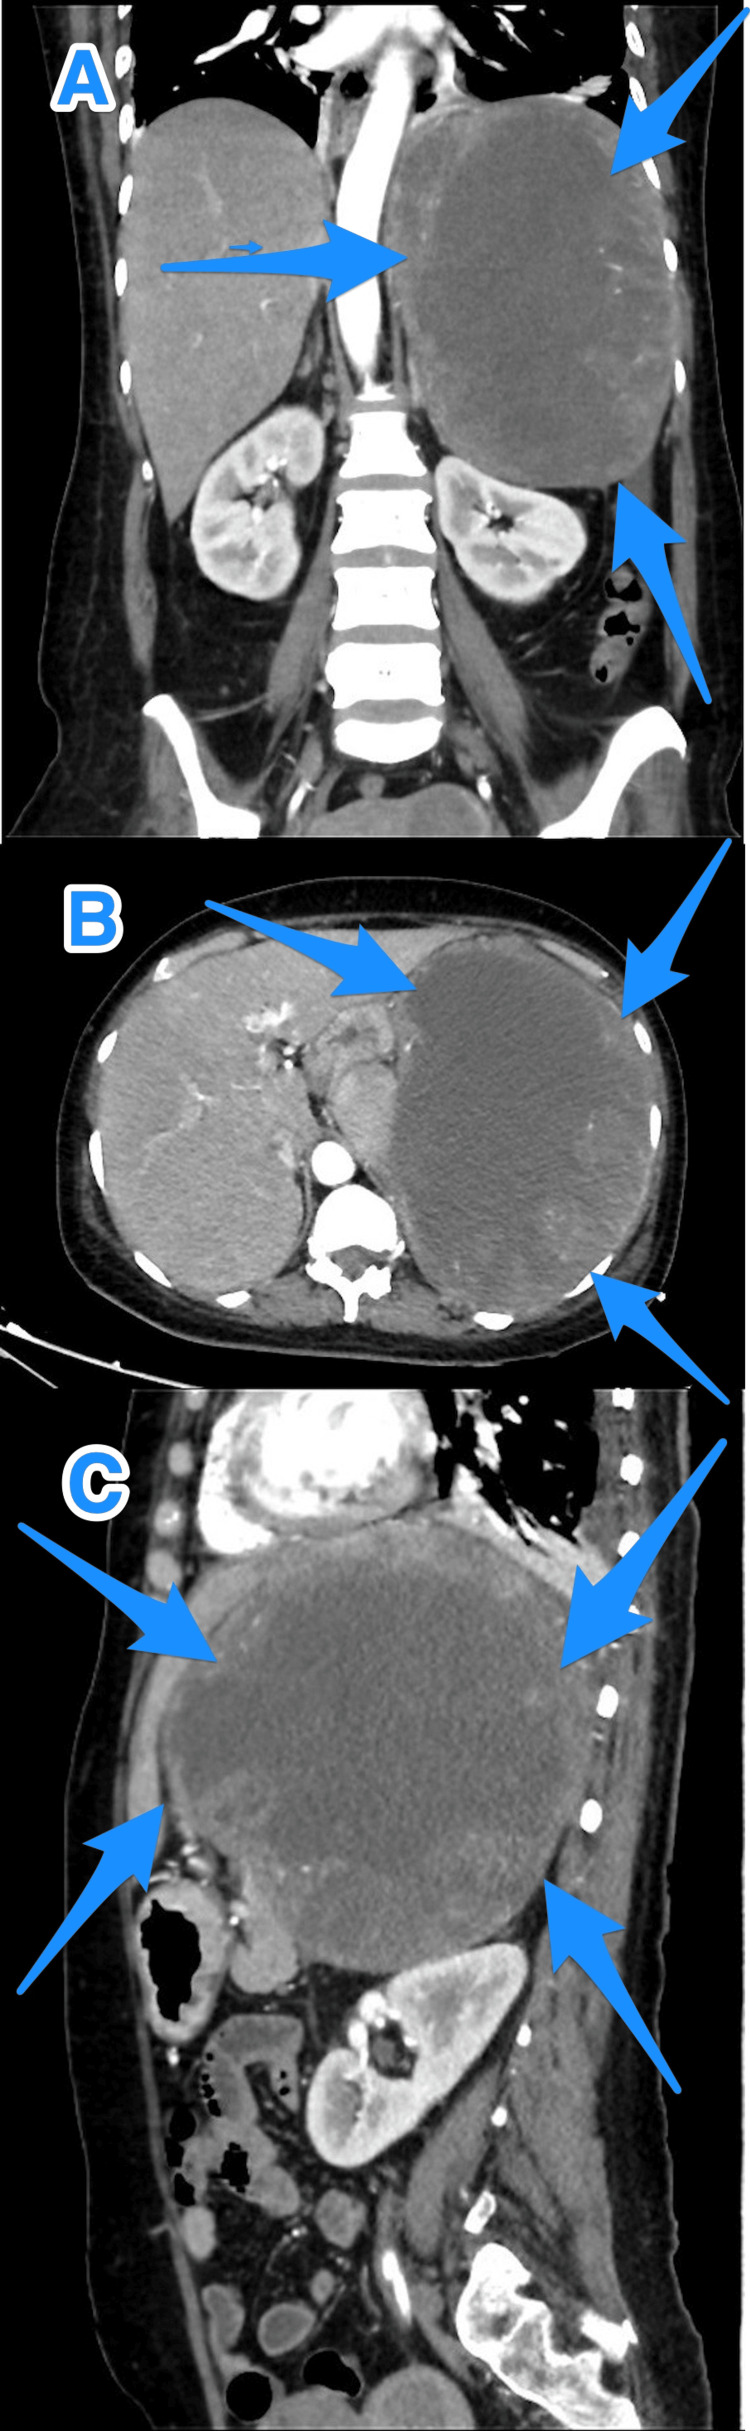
A multiview computed tomography scan of the abdomen (A-C) showing a large mass occupying the left upper quadrant of the abdomen, with no clear origin or borders between the pancreas, spleen, and stomach (arrows).
